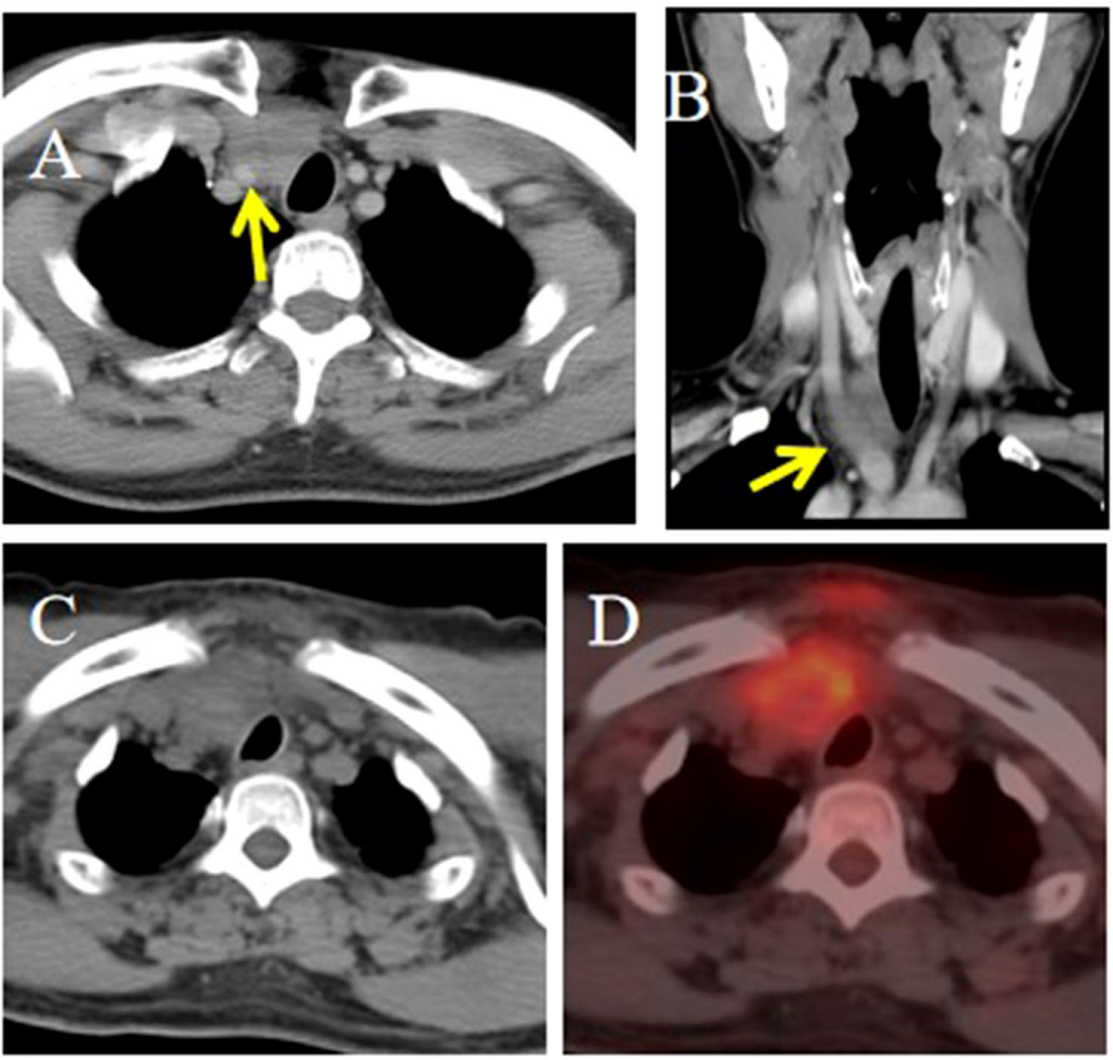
CT and PET/CT imaging of the ITTC. (A, B) Tumor located in the right lower pole of the thyroid and right suprasternal fossa; the tumor was inseparable from the right common carotid artery at multiplanar reconstruction (yellow arrow). (C, D) It demonstrated obvious focal FDG tumor uptake.
